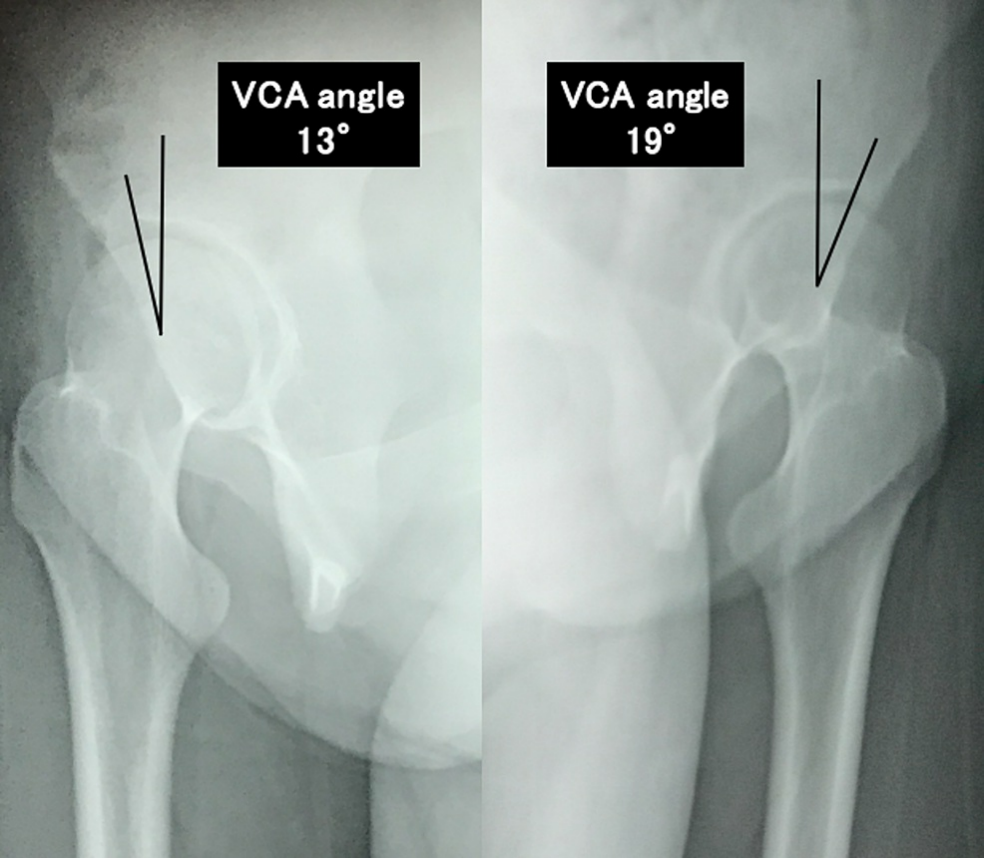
Radiograph, false profile view, shows bilateral acetabular dysplasia of the hip. VCA: vertical center anterior
